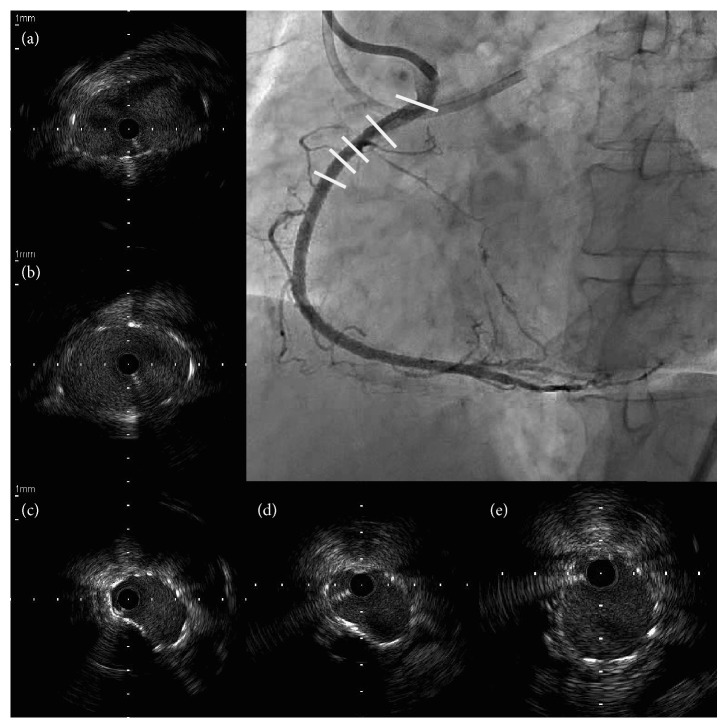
A final angiogram reveals an acceptable outcome. Serial intravascular ultrasound images of right coronary artery (RCA) after stenting show the ostial RCA (a), RCA proximal to chronic total occlusion (CTO) (b), and the CTO segments ((c)–(e)). Images are viewed in a proximal (a) to distal (e) direction. Adequate stent expansion is achieved throughout the lesion, except elliptical stent expansion limited by a superficial calcified lesion at the calcified proximal cap of CTO (c).
